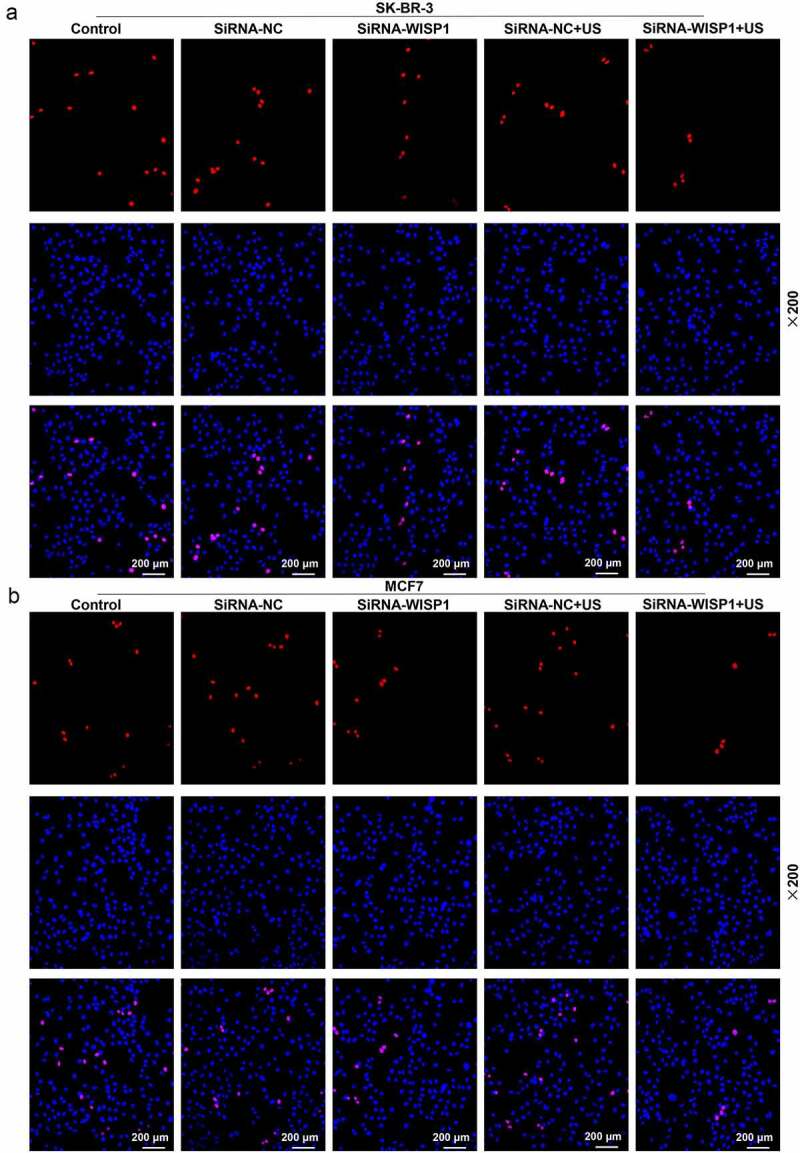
Effects of siWISP1 transfection mediated by US or Lipofectamine 6000 on breast cancer cell proliferation. (a-b) EdU assay was used to detect the cell proliferation in the control, siRNA-NC, siRNA-WISP1, siRNA-NC+US and siRNA-WISP1+ US groups (magnification × 200). Scale bar = 200 μm. Abbreviation: siWISP1, WNT1 inducible signaling pathway protein 1 small interference RNA; US, ultrasonic irradiation and SonoVue microbubbles; NC, negative control.
